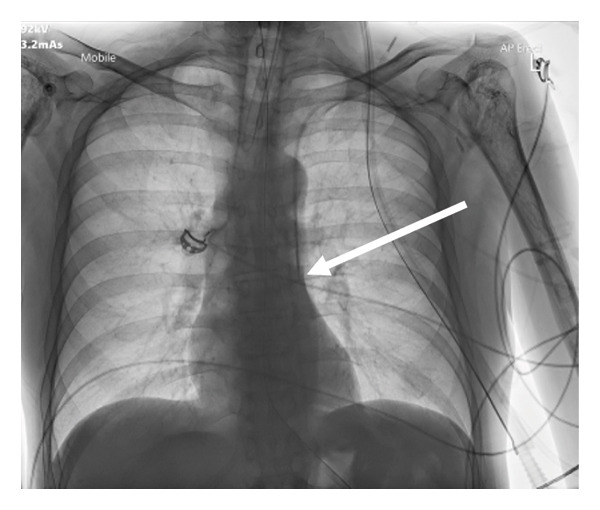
Chest X‐ray demonstrating catheter misplacement. Chest X‐ray shows the central venous catheter coursing along the left mediastinum (white arrows), raising suspicion of placement in the left superior vena cava (PLSVC). Prior CT imaging from 2019 (Figures 2 and 3) confirmed the presence of this anomaly.
